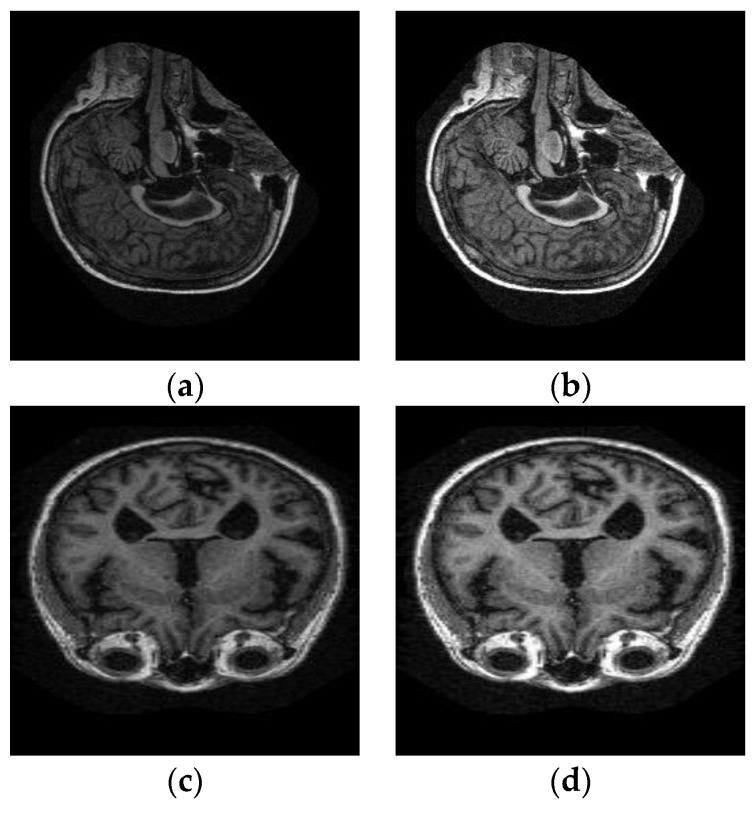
(a,c) Input MRI scans. (b,d) Contrast Stretched output Images.
